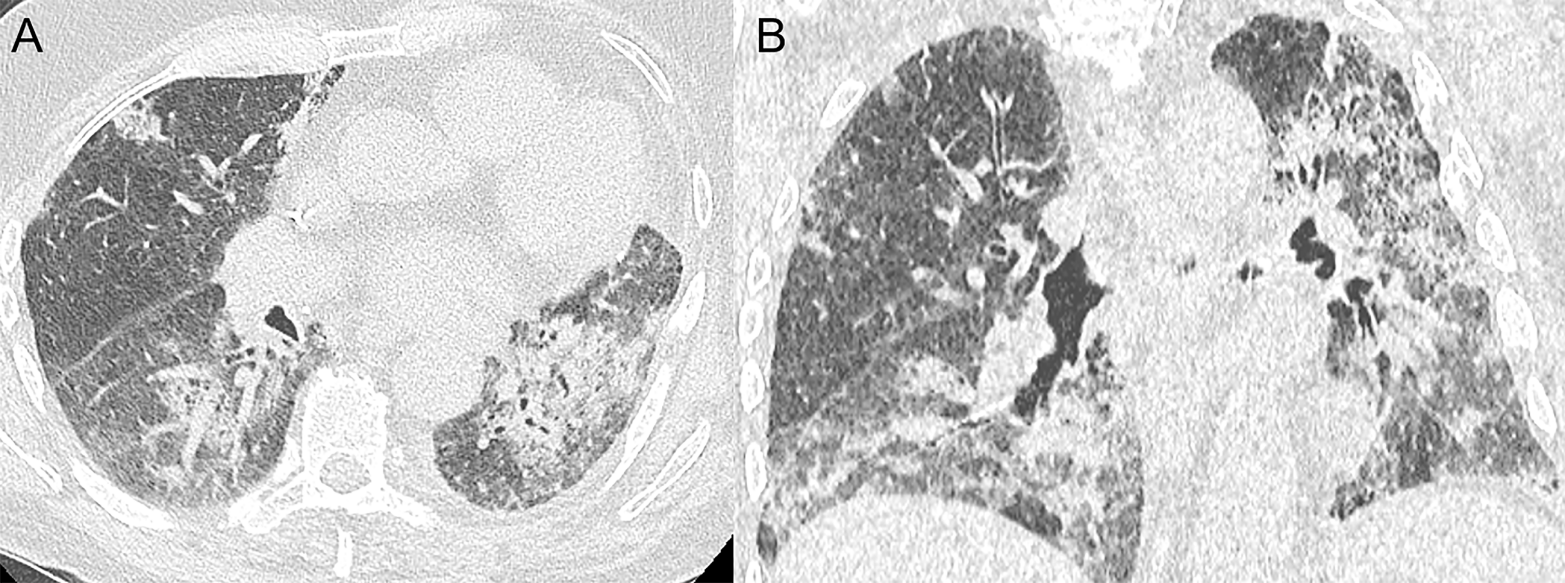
Immune-related pneumonitis in a 79-year-old male with stage IV non-small cell lung cancer in the left lower lobe. After second line treatment with nivolumab (anti-PD-1) (5 cycles), the patient developed progressive dyspnea and dry cough. Axial (A) and coronal (B) CT images demonstrate multifocal alveolar consolidations in a predominantly peribronchovascular and subpleural location compatible with a drug-induced pneumonitis.
